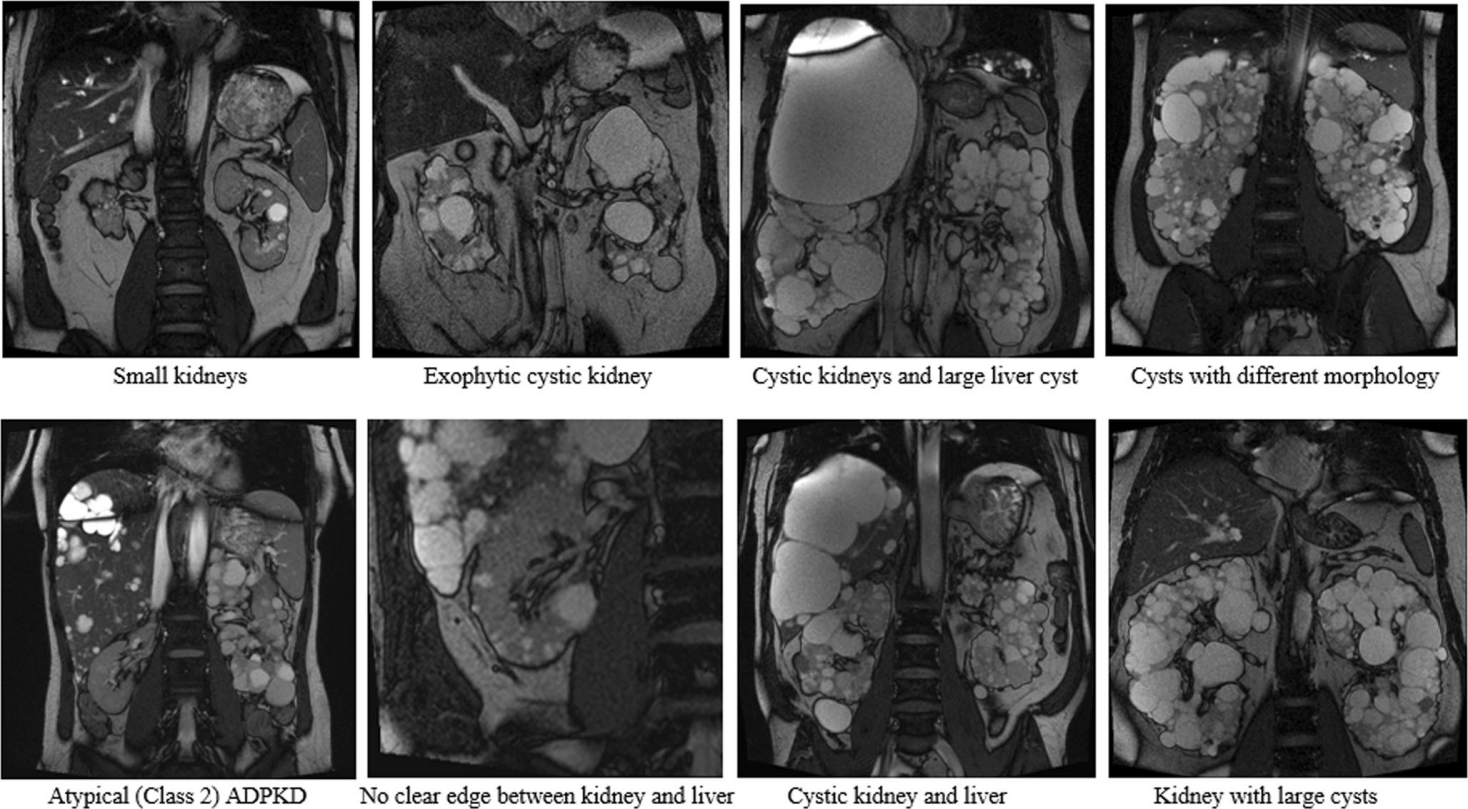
Representative ADPKD kidney MR images used to test Sheffield TKV Tool
